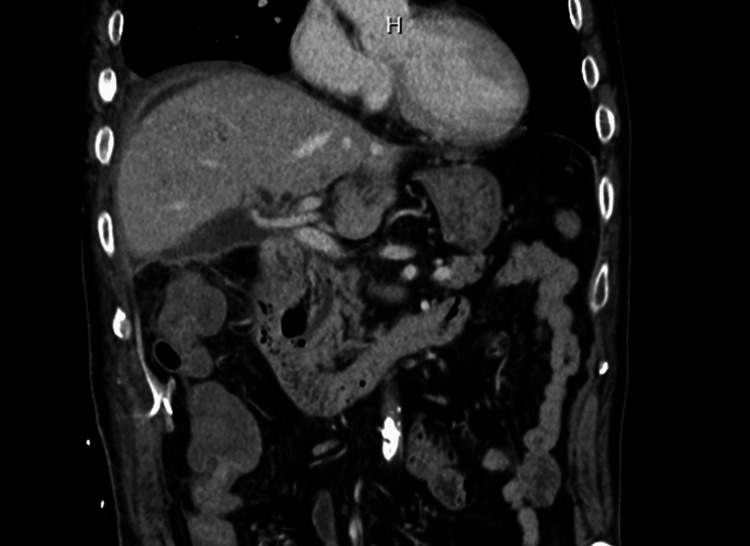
CT before reoperation showing disconnection of the common bile duct and dilation of the intrahepatic bile duct
